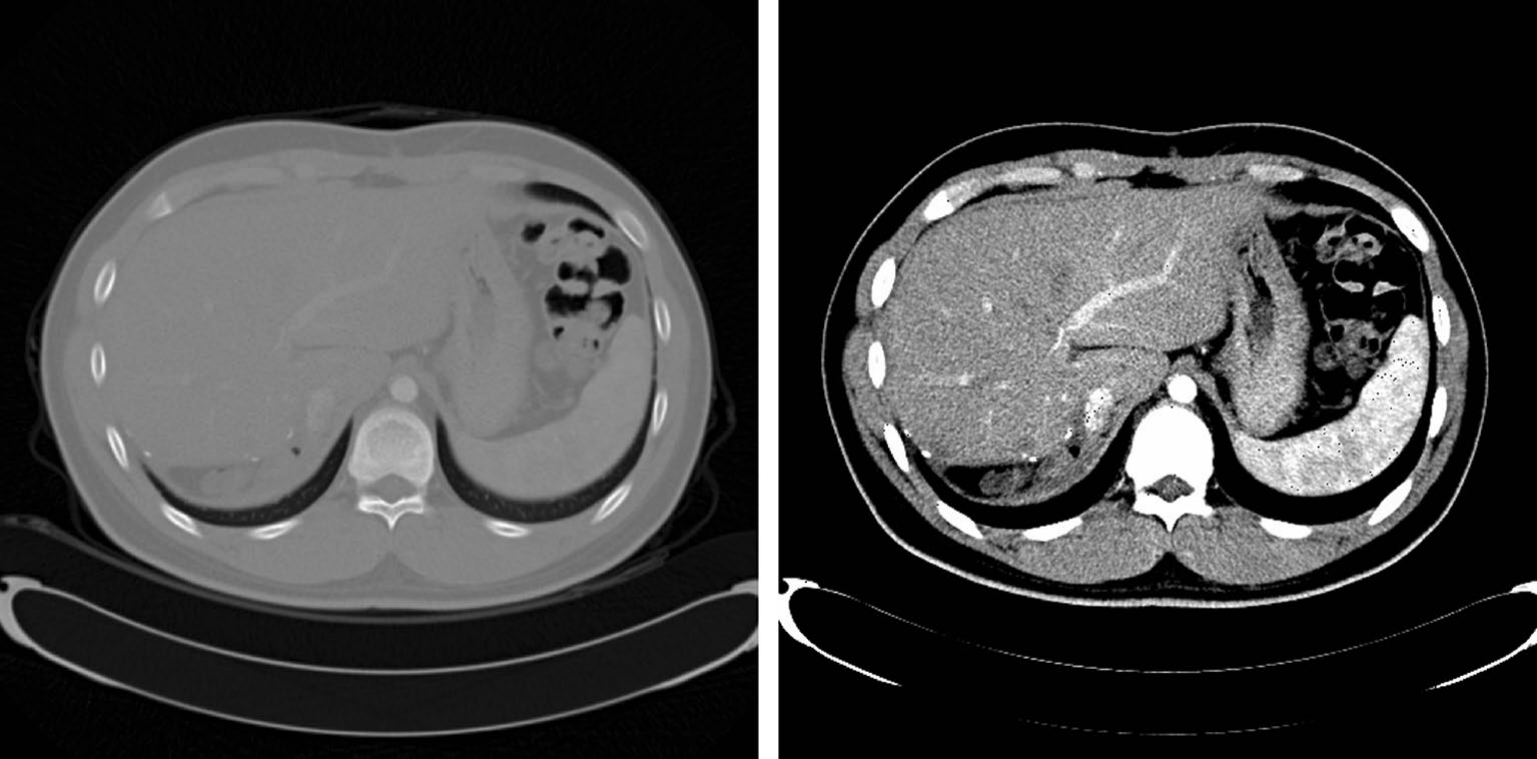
Comparison between the raw computed tomography axial view images (left) and with the window setting (right).
